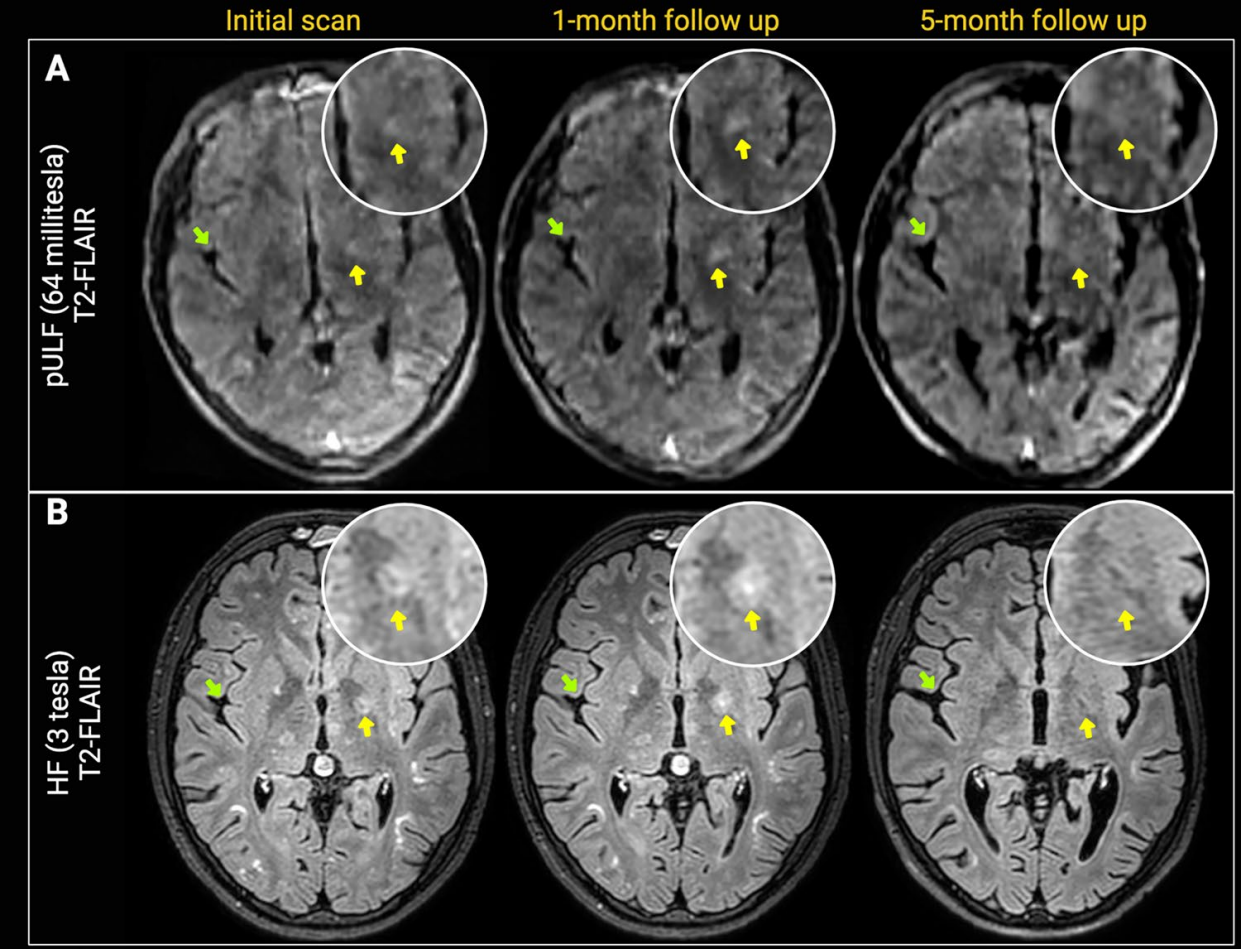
pULF-MRI shows PML lesion progression and regression over time. 21-year-old man with PML and underlying primary immune deficiency. T2-FLAIR images acquired with pULF-MRI (64 miliTesla) (panel A) and HF-MRI (3 T) (panel B) are shown. pULF-MRI and HF-MRI were acquired within the same week in the initial scan, 1-month and 5-month follow up scans. PML lesion extending to the right deep gray matter area becomes more conspicuous in the 1-month follow up, and regression of this lesion is apparent on 5-month follow-up scans. These changes are visualized in both pULF-MRI and HF-MRI (yellow arrows, magnified view). Enlargement of the CSF spaces and Sylvian fissures (green arrows) suggesting parenchymal volume decrease is visualized on pULF-MRI during the follow-up. Arrows suggesting parenchymal volume decrease is visualized on pULF-MRI during the follow-up
